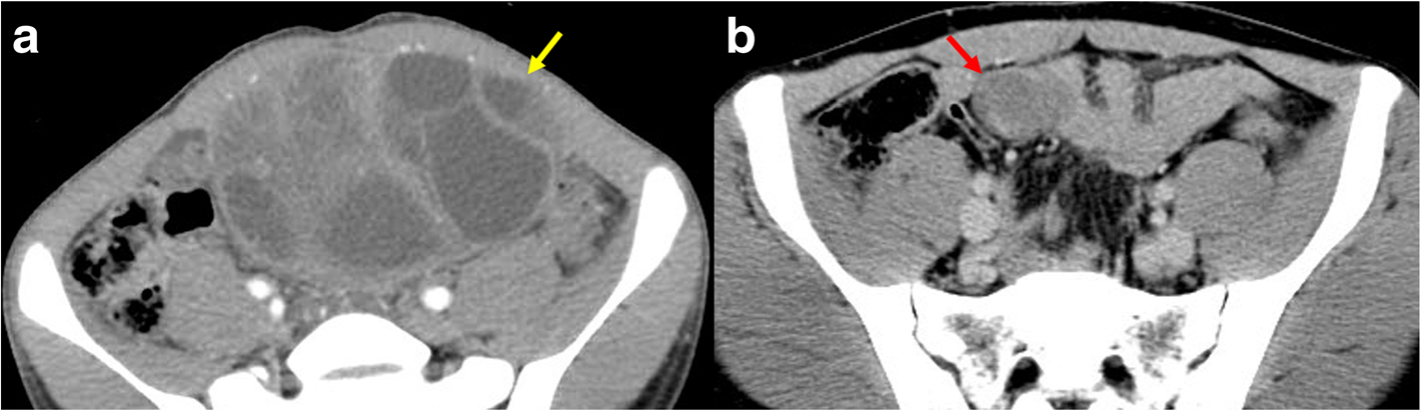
CT images for patient’s history of spindle cell sarcoma (SCS), originating from lower abdominal wall and 1st peritoneal recurrence after surgery. a Very large SCS stands out from lower abdominal wall (*yellow arrow*) when the patient was 13 years old. b Peritoneal recurrence of SCS on the mesentery over the small intestine (*red arrow*) when the patient was 22 years of age. Both lesions were curatively resected
